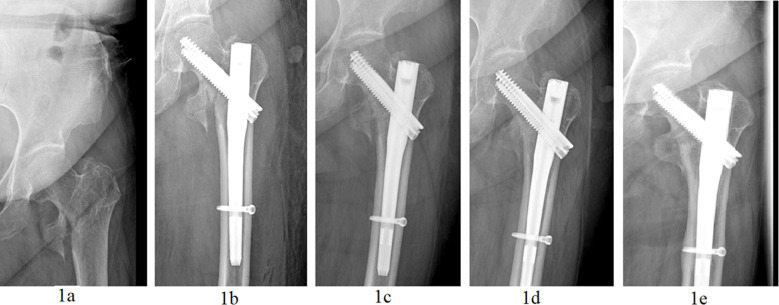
InterTAN internal fixation in a 71-year-old female patient with a left intertrochanteric fracture. 1a: X-ray image showing intertrochanteric fracture of the femur after the injury. 1b: X-ray image immediately after the operation showing appropriate fracture reduction. 1c: X-ray image showing appropriate internal fixation evolution one month after the operation. 1d: X-ray image showing bone union at the fracture site six months after the operation. 1e: X-ray image showing appropriate fracture healing 12 months after the operation.
